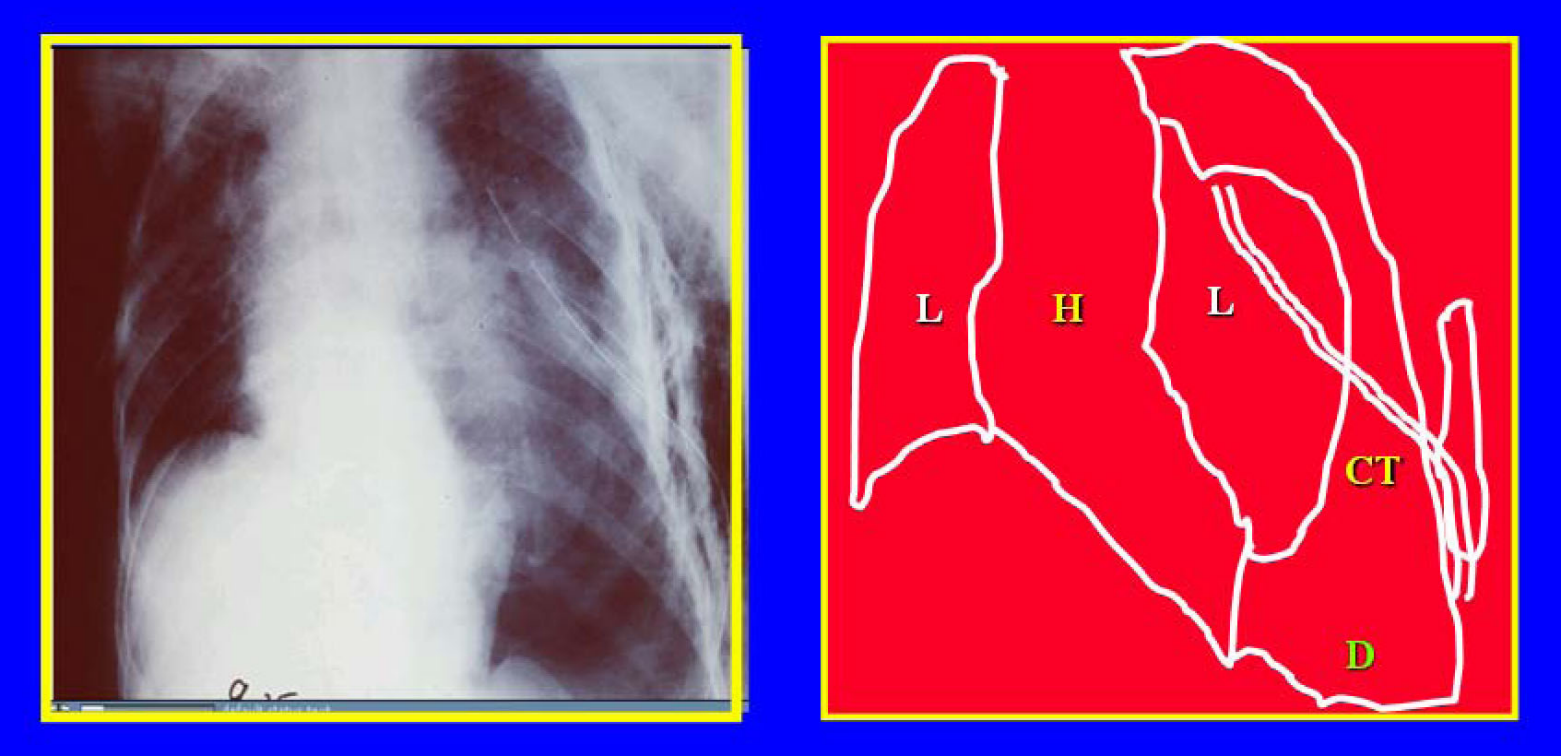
A 45-years old male who developed severe hypoxia while being ventilated despite having a chest tube. L = lung, H = heart, CT = chest tube, D = diaphragm.
